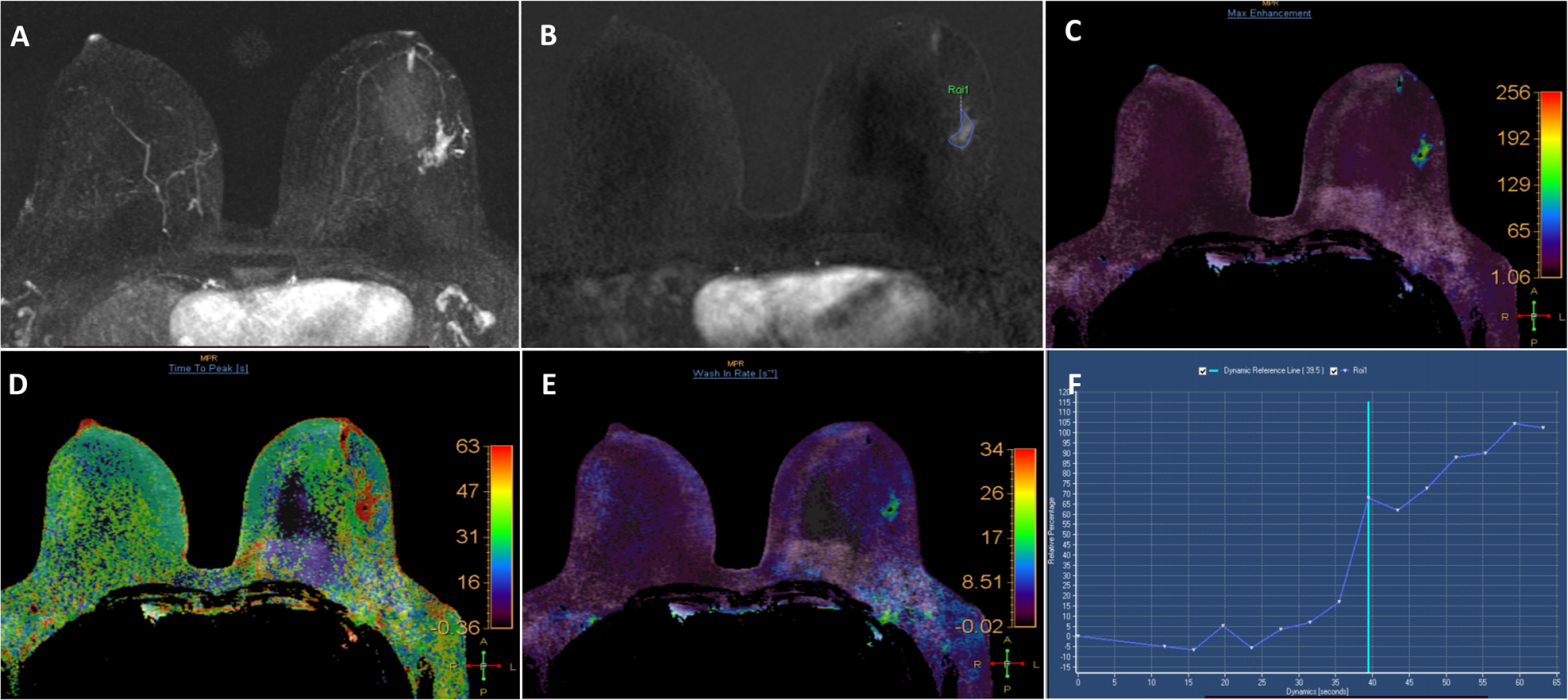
A 32-year-old female presented with a left breast mass (A), subtracted MIP image derived from ultrafast MRI at phase 13, showing an irregular mass with spiculated margin in the left lower outer quadrant. B Subtracted ultrafast image at the same phase showing freehand ROI outlining the lesion. C–E Semiautomatically generated color-coded maps for ME, TTP, and wash-in rate showing the previously described mass displaying different colors from the rest of the normal breast tissue. F The wash-in curve was generated once the ROI was placed on the lesion
